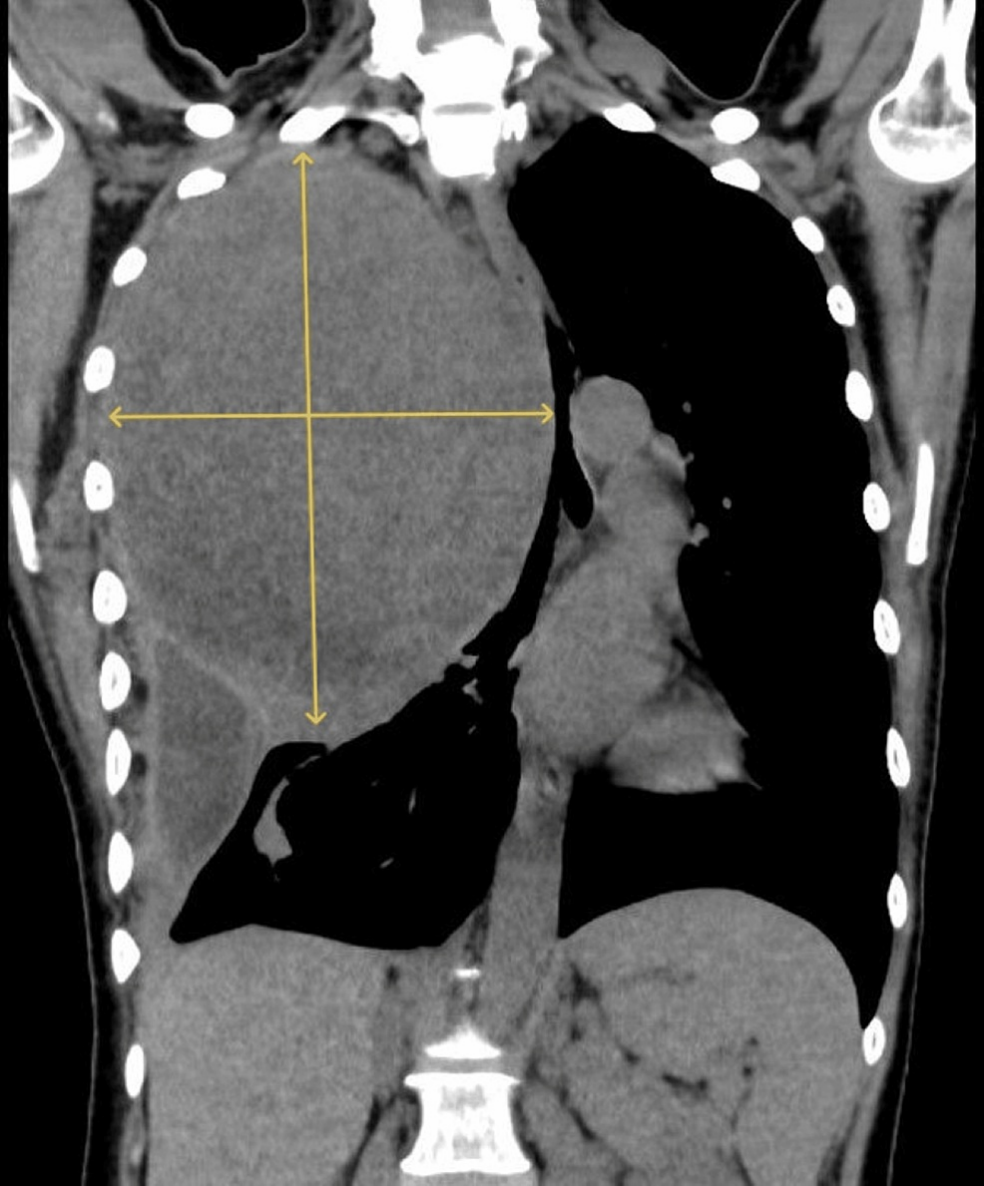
Contrast-enhanced CT of the chest at admission (coronal image) Three-dimensional CT of the trachea and bronchi. The right lung and trachea are compressed by a large mass (a chest tumor measuring 135x175mm). CT, computed tomography
